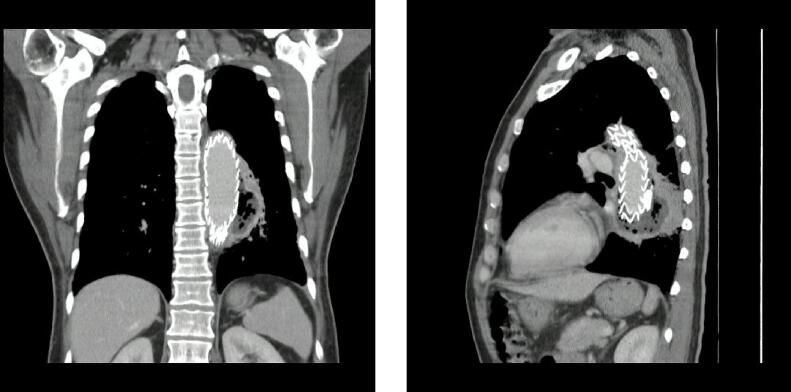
CT angiography with periprotesic gas suggestive of periprotesic infection.
